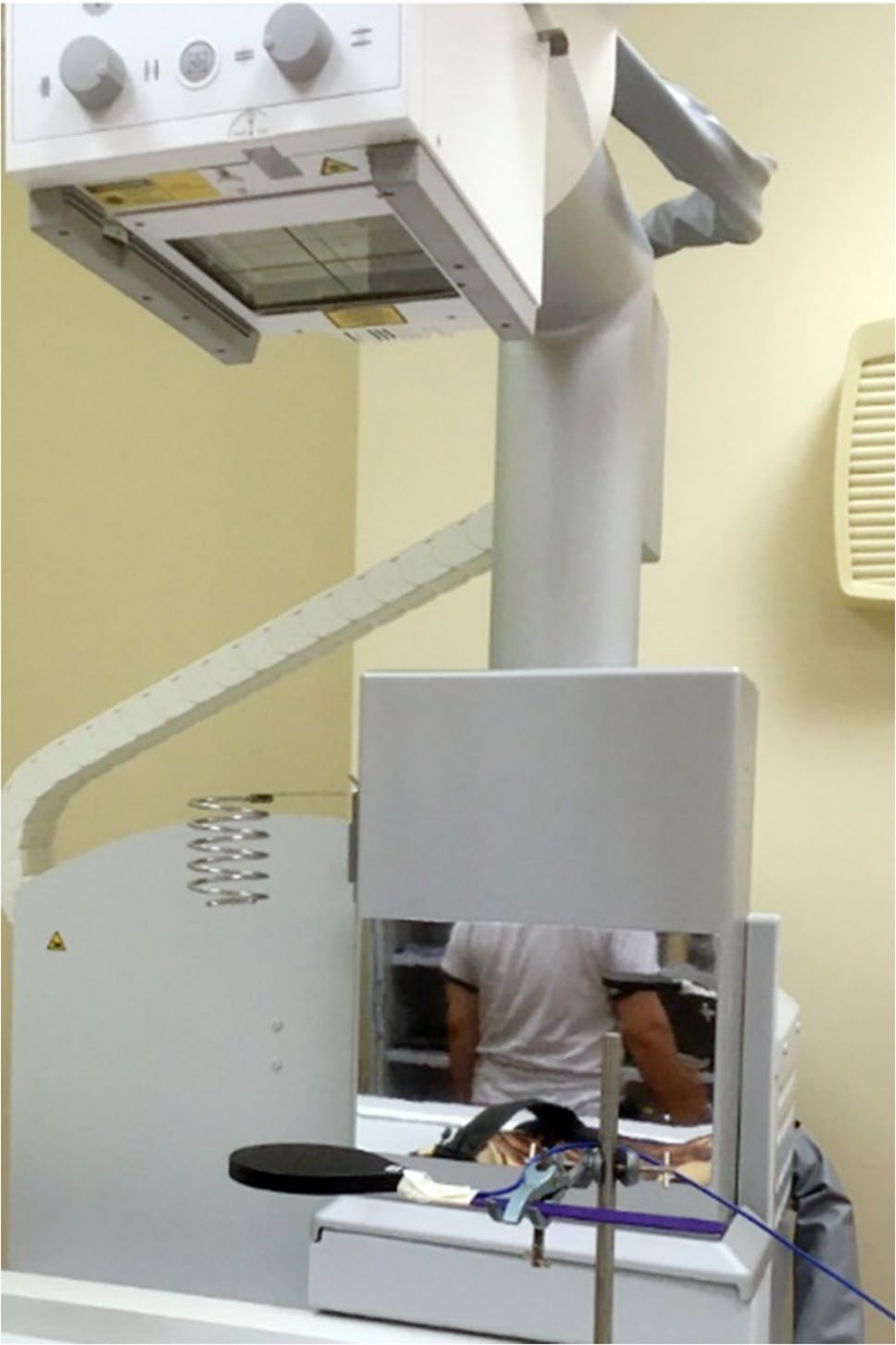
A photograph shows  a Siemens dRF radiographic unit and the 75-cm^3^ pancake chamber
